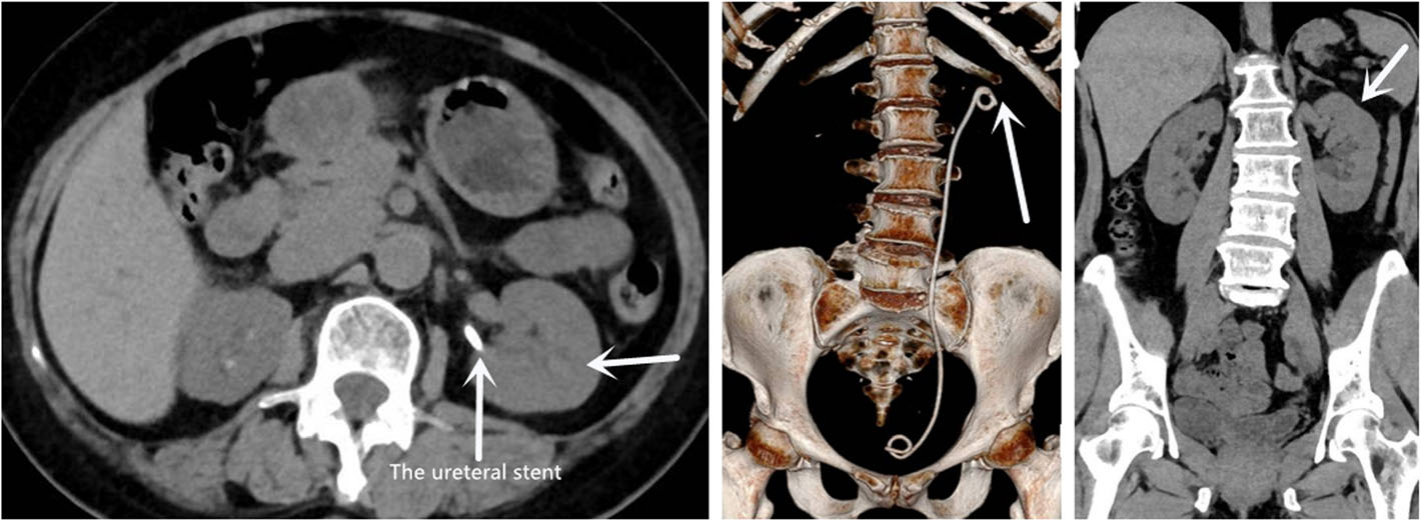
There were no stone residents observed on CT scan 1 month later postoperatively
